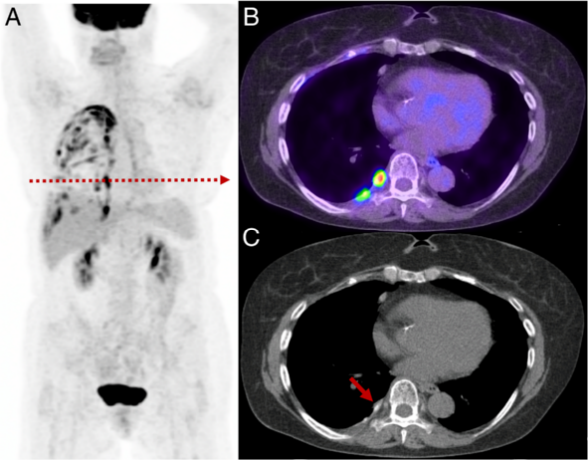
Patient with prior lung malignancy presents for surveillance. The study demonstrates a typical appearance of inflammatory change post talc pleurodesis with intense multi-focal uptake evident throughout the pleural surface (a). On the axial PET/CT (b) and CT (c) the high focal uptake correlates with a site of talc on CT recognised by its high density. Such change can persistent for many years after pleurodesis
